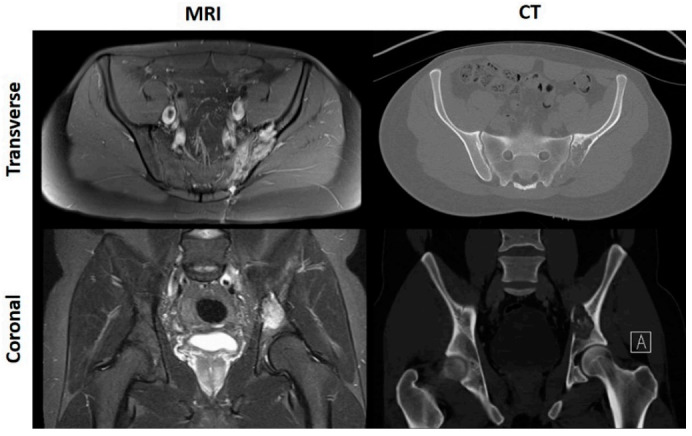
MRI and CT scans of a patient with left sided pelvic OS.
